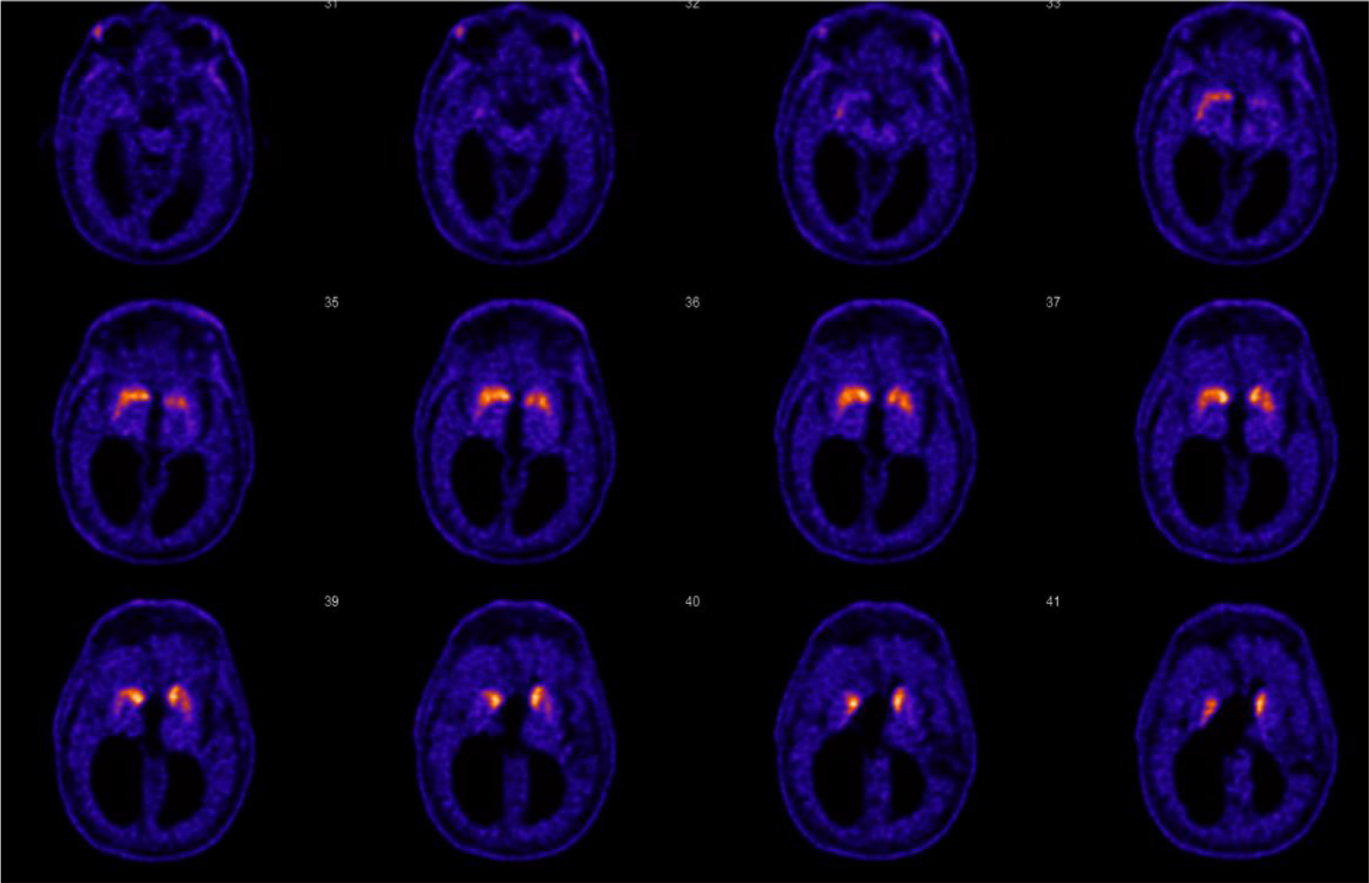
F18-FE-PE2I striatum brain PET-CT with signs of degeneration of the presynaptic nigrostriatal dopaminergic pathways were described, as in idiopathic Parkinson's disease or Parkinson-plus syndrome with left-sided predominance.
